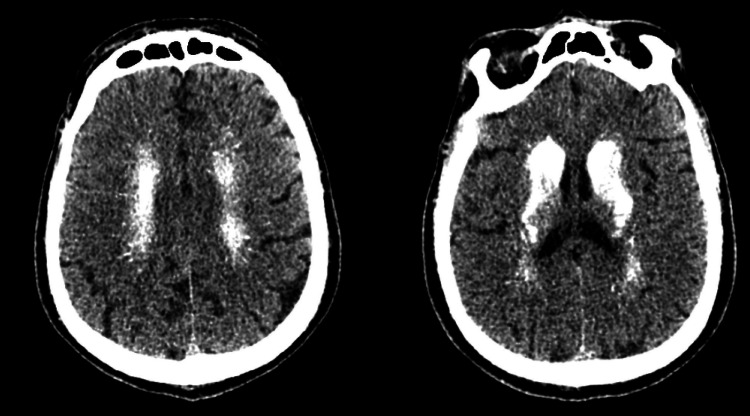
Cranial CT demonstrating important calcification in subcortical tissues.
